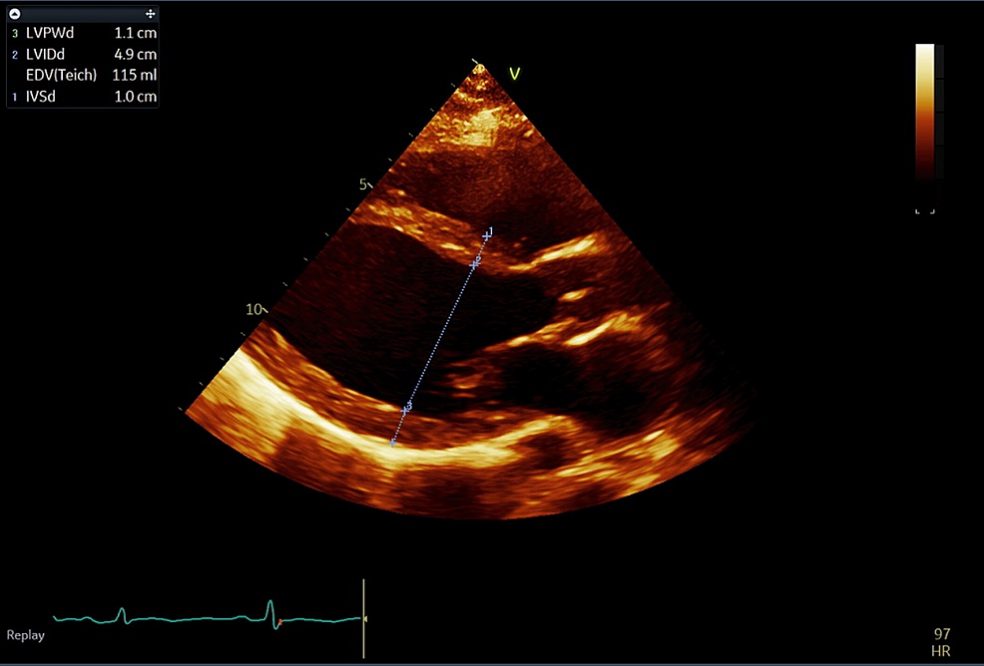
Parasternal long axis on transthoracic echocardiogram at end-diastole demonstrating mild left ventricular hypertrophy.
